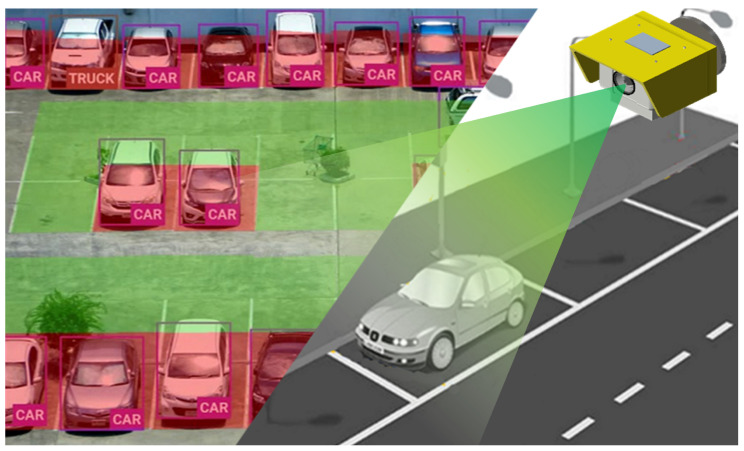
Installation example and structural details of an ultrasonic sensor-based parking detection system.
